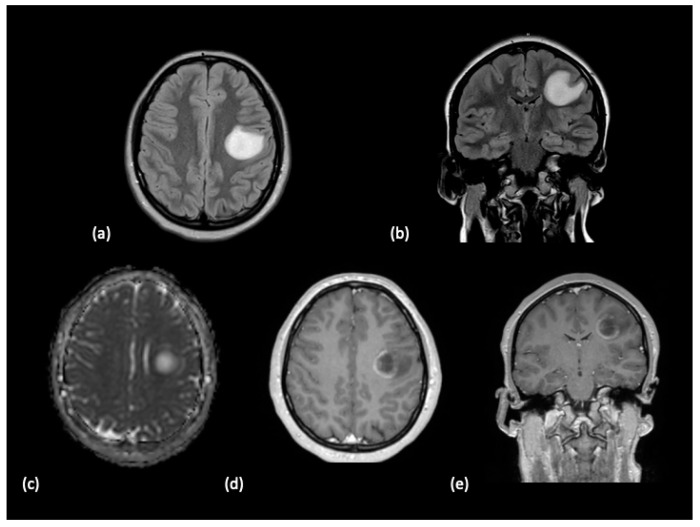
A 15-year-old female with a history of bilateral optic neuritis presented with stroke-like symptoms including facial droop and slurred speech. (a) Axial and (b) coronal FLAIR sequences demonstrated a large hyperintense lesion located in the left middle frontal lobes, precentral gyrus and the frontal operculum. (c) The lesion exhibits mild diffusion restriction at the periphery. Partial peripheral enhancement along the medial margin of the lesion was noted on the (d) axial and (e) coronal post-contrast T1-weighted images. The patient history and imaging features over time supported a diagnosis of tumefactive multiple sclerosis.
